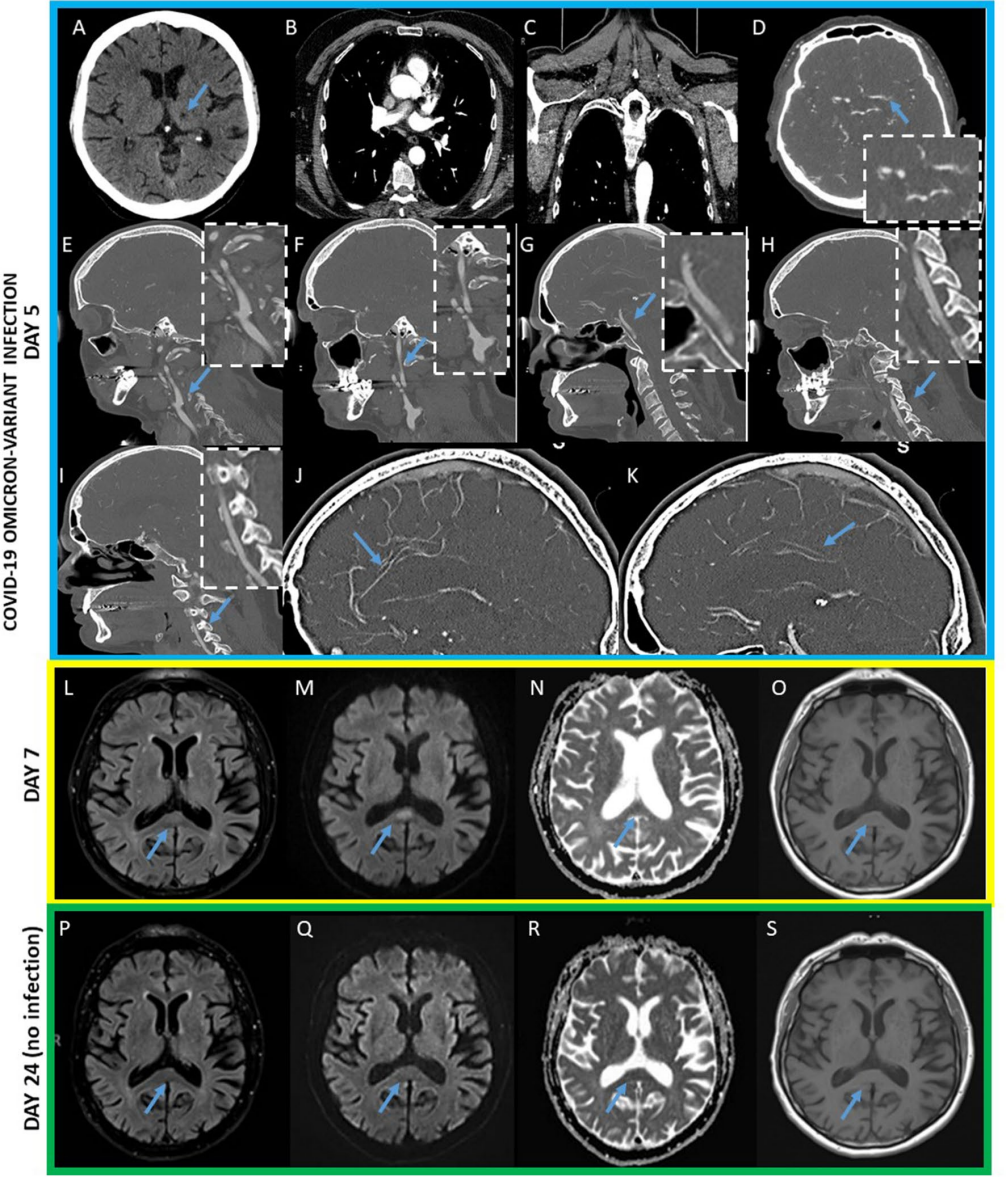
A-S Neuroradiological features detected in the patient described. A–K CT-Angiography of thorax, head and neck, 5 days post COVID-19 Omicron infection. A Axial-CT-Scan displaying an old left thalamic infarct (blue arrow). B-C Thorax-CT axial and coronal scans, without suggestive features for a COVID-19 Omicron pneumonia. D-K CT-Angiography of head and neck without vessel stenosis or occlusion and nor neuroradiological hallmarks typical for a vasculitis (blue arrows). D Circle of Willis (blue arrow). E Left extracranial carotid artery (blue arrow). F right extracranial carotid artery (blue arrow). G Arteria basilaris (blue arrow). H Left arteria vertebralis (blue arrow). I Right arteria vertebralis (blue arrow). J Anterior pericallosal artery (blue arrow). K Posterior pericallosal artery (blue arrow). L–O axial MRI scans performed 7 days post COVID-19 Omicron infection displaying a round midline lesion in the splenium of the corpus callosum with subtle hyperintensity on fluid attenuated inversion recovery (FLAIR) (L) corresponding to a T2-weighted imaging with restricted diffusion (of ca. 5 x 12 mm) (M) and low signal intensity on apparent diffusion coefficient (ADC) (N) and a very mild hypointense signal on T1-weighted imaging (O) (blue arrows). P–S, axial control MRI scans performed 24 days post COVID-19 Omicron infection presenting a complete resolution of the lesion in the splenium of the corpus callosum in the T2-FLAIR sequences (P) as well as in the T2- Diffusion*-*weighted imaging (DWI) (Q), on apparent diffusion coefficient (ADC) sequences (R) and on T1-weigted imaging (S) (blue arrows), patient asymptomatic and SARS-CoV-2 Rapid Antigen Test negative
